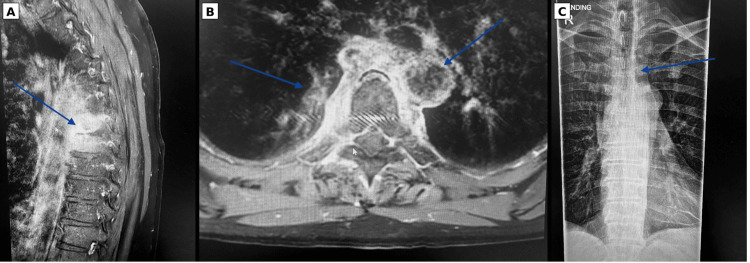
MRI & X-ray Additional MRI findings and follow-up imaging. (A) Post-contrast sagittal thoracic MRI with fat saturation. A strip of enhancement is seen anterior to the vertebral column from approximately T5/T6 to T9, in keeping with periaortic inflammatory change. This was not apparent on the CT angiography performed the previous day. (B) Axial post-contrast image at the level of the affected segment, with prevertebral soft tissue involvement abutting the oesophagus and azygous vein. There was no epidural extension or cord compression. (C) Thoracic spine anteroposterior radiography, 28 January 2026. Persistent T6–T7 disc space narrowing with endplate erosion, with reduced vertebral body heights at T6, T7, and T8. Spinal alignment was preserved and there was no vertebral collapse.
